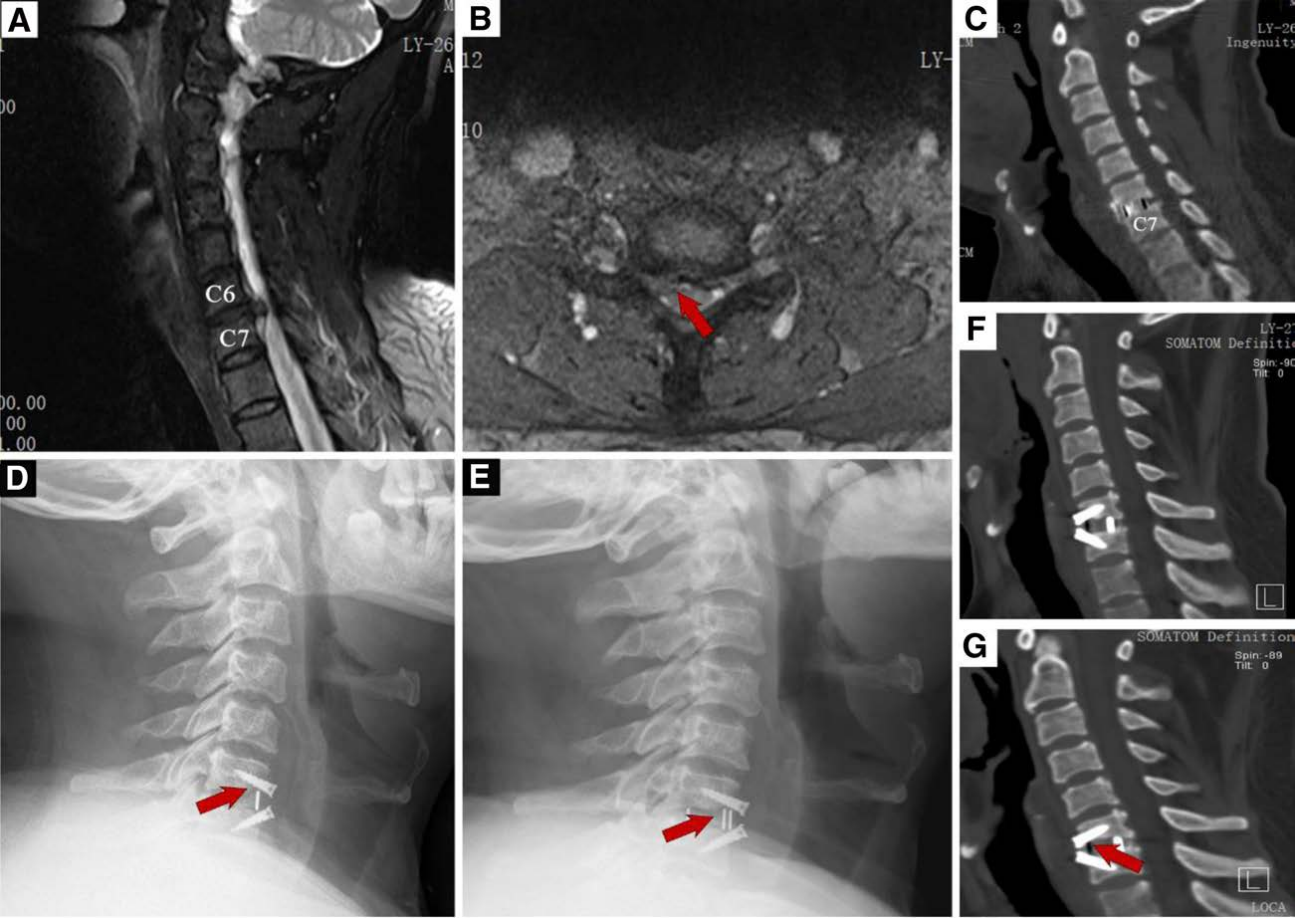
The radiology of patients was followed up before and after operation. (A–B) Preoperative magnetic resonance imaging. (C) CT imaging three days after operation. (D–E) The X-ray films of 1 month and 2 months after operation, bone resorption was indicated by red arrow. (F–G) CT three months after operation showed obvious signs of bone resorption. CT = computed tomography.
